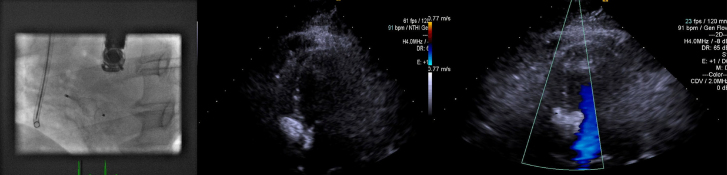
(top left) Fluoroscopic image demonstrates the confirmed position of the device, (top right) transthoracic echocardiogram (TTE) demonstrates the confirmed position of the device, (bottom middle) postoperative 30th day control TTE demonstrates the successful closure.
